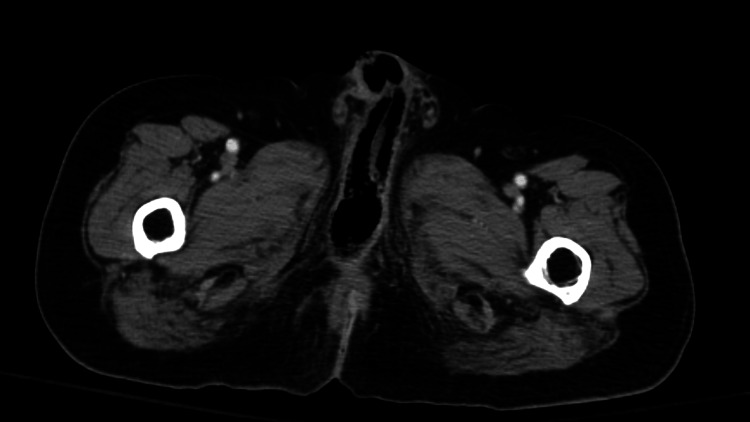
Contrast-enhanced CT axial image of the pelvis demonstrated air within the corpus cavernosum There were inflammatory changes in the fat and soft tissues of the perineum.
